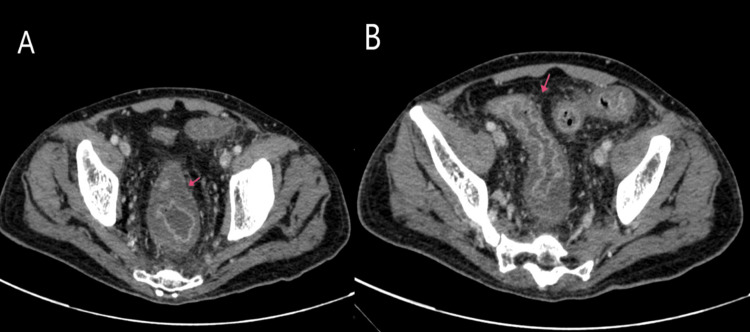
Computed Tomography (CT) showing mural thickening with peri-colic fat stranding (Red arrow) involving rectum (A) and sigmoid colon (B)
